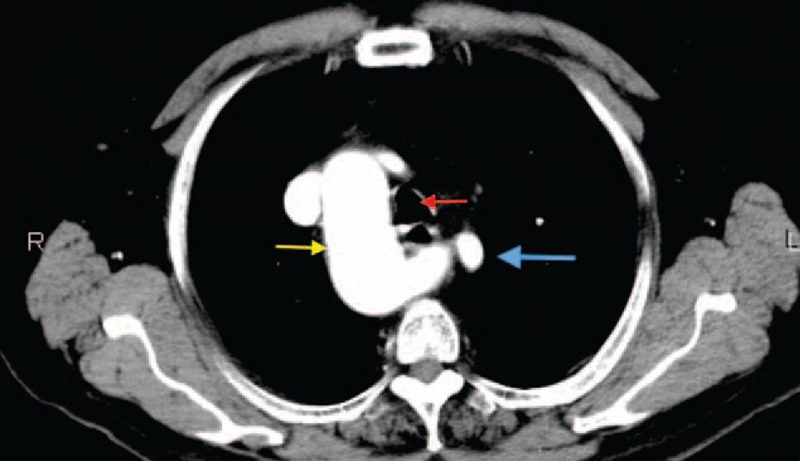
CT scan image. Axial view. RALS. (Blue arrow, aberrant left subclavian artery; red arrow, trachea; yellow arrow, right aortic arch.) CT = computed tomography, RALS = right aortic arch with aberrant left subclavian artery.
